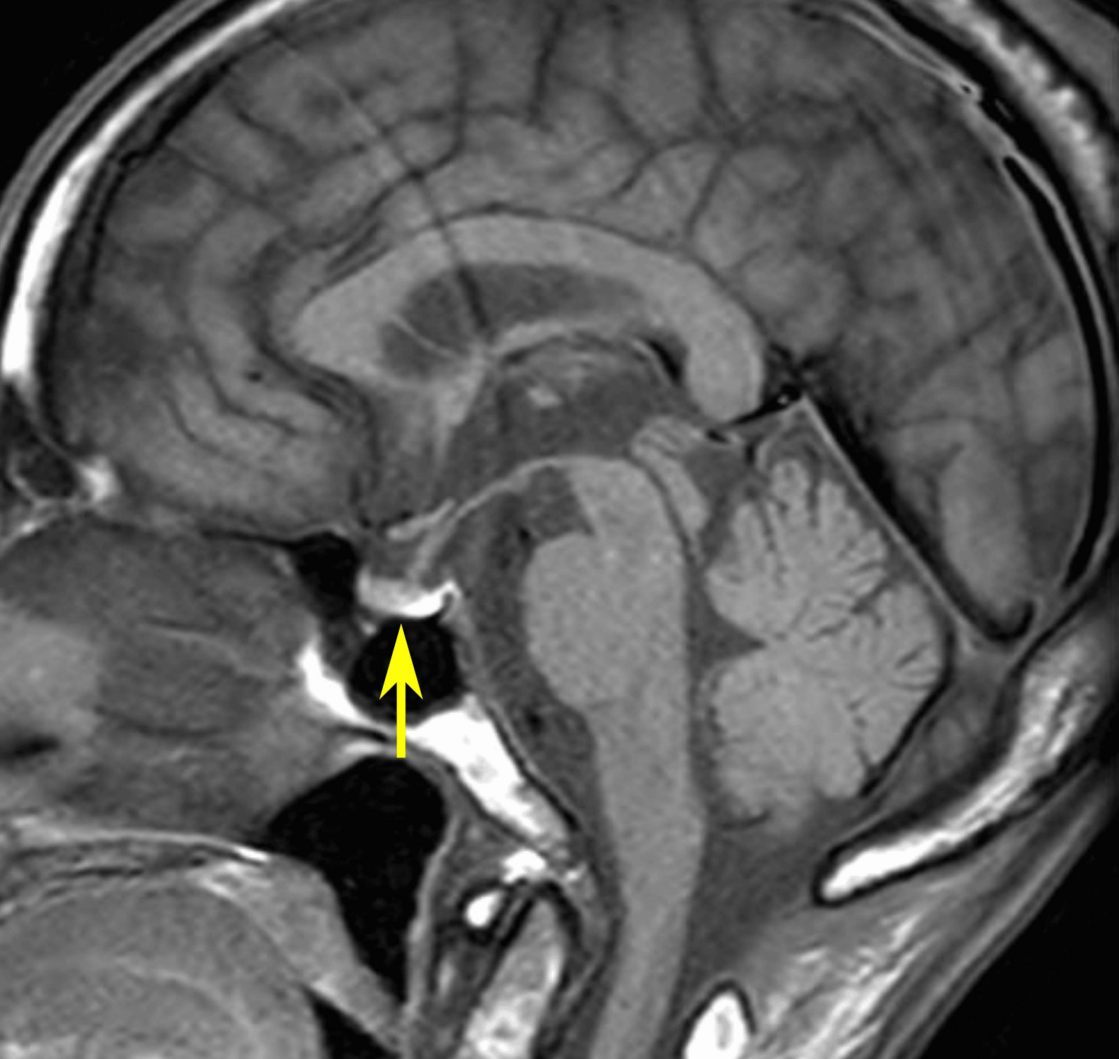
Brain MRI performed in June 2023. Sagittal T1-weighted plain MRI showed no abnormality in the pituitary gland (yellow arrow). MRI: magnetic resonance imaging.
